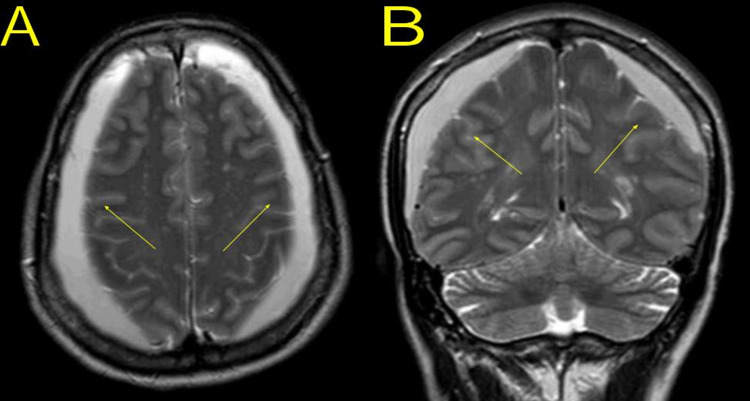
Brain magnetic resonance imaging showing the bilateral chronic subdural hematoma (yellow arrows): (A) axial T2-weighted image, (B) coronal T2-weighted image.
